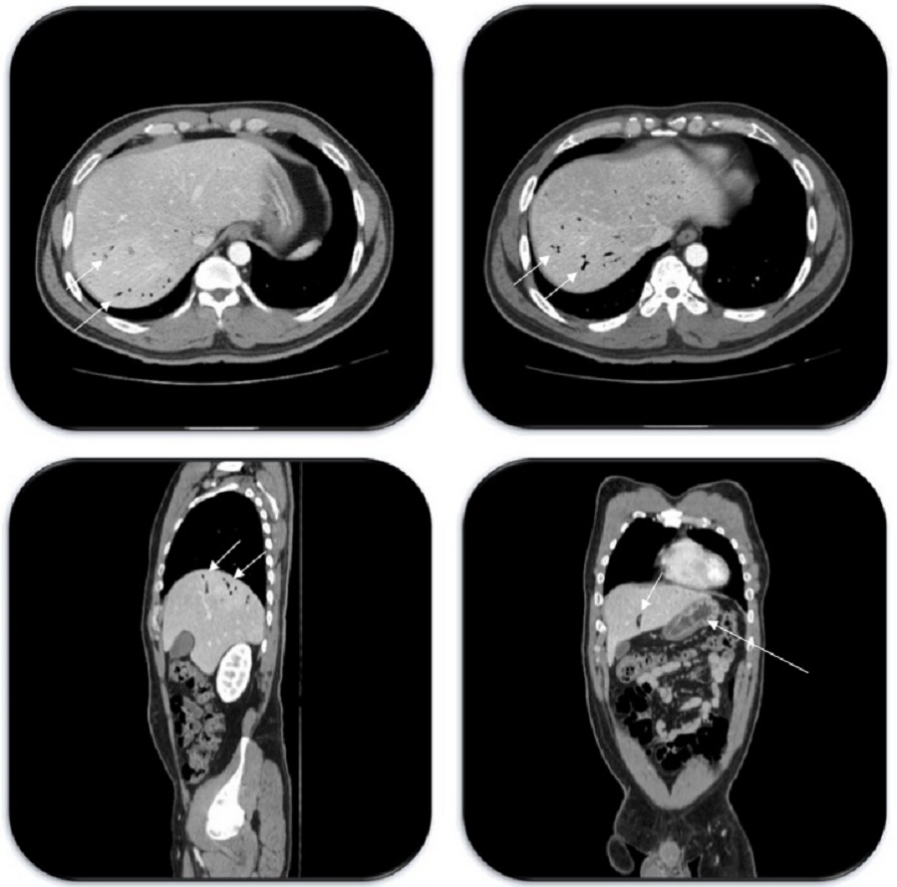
Axial (upper two images), sagittal (left lower image), and coronal (right lower image) abdominal CT scan images showing diffuse portal venous gas (short arrows) and gastric wall thickening (long arrow) up to 1 cm CT: computed tomography
